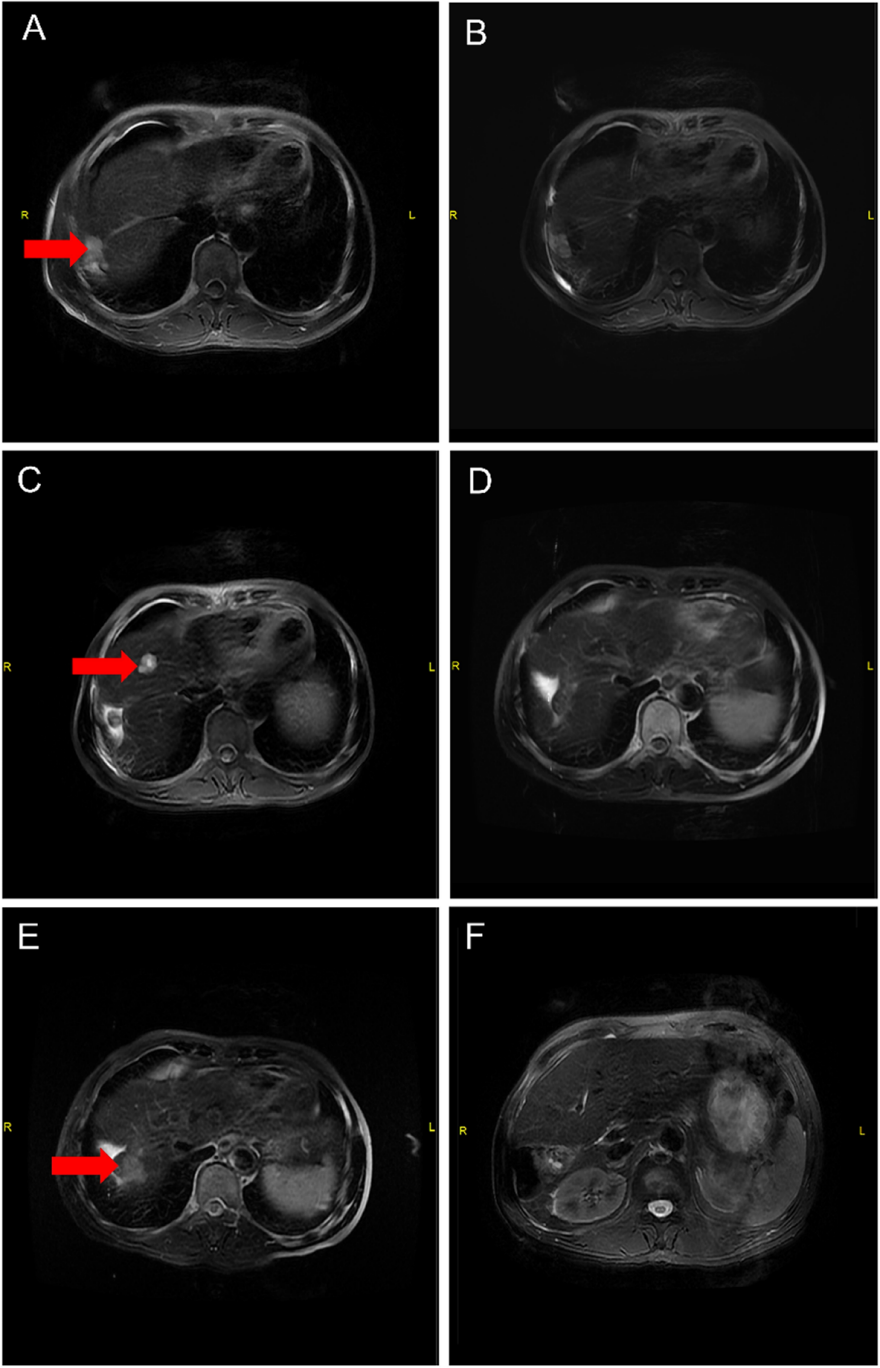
The patient who received three courses CK-SBRT. November 2014: The primary abdominal MRI scan with the HCC lesion (in right liver) (a). Then the patient received CK-SBRT with 56Gy/7f. July 2015: MRI scan of 8 months after CK-SBRT (lesion in right liver) (b). The activity of lesion had disappeared. June 2016: MRI scan of lesion right liver after CK-SBRT; New lesion occurred in liver, thereafter the patient received second-course CK-SBRT with 54Gy/6f (c). February 2017: MRI scan of first-treated and second-treated lesion (d). The activity of two lesions had disappeared. November 2017: There was an activity tumor adjacent to first-treated lesion, thereafter the patient underwent 49Gy/7f of CK-SBRT (e). July 2019: MRI scan of the treated area after CK-SBRT (f)
